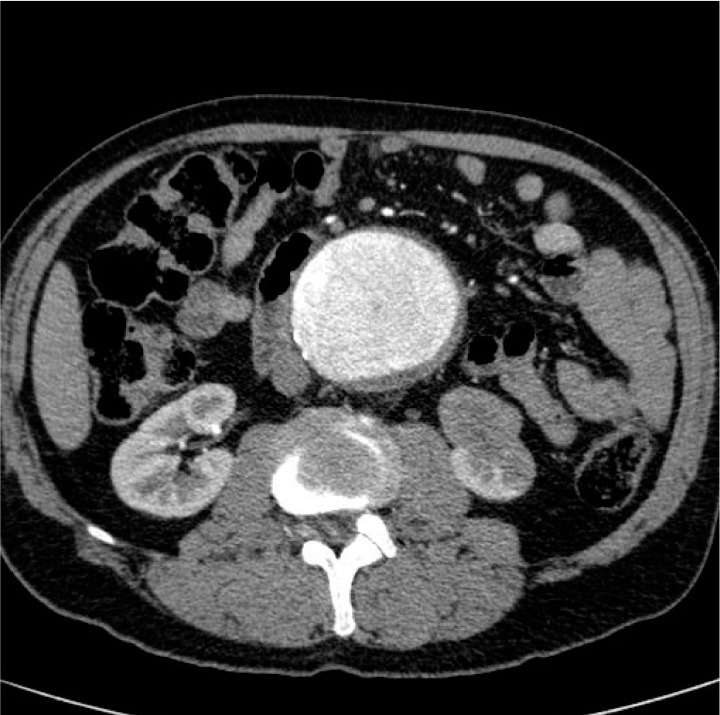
Computed tomography of abdomen demonstrating left-sided lower pole renal tumour (highly suspicious of renal cell carcinoma) together with large abdominal aortic aneurysm
